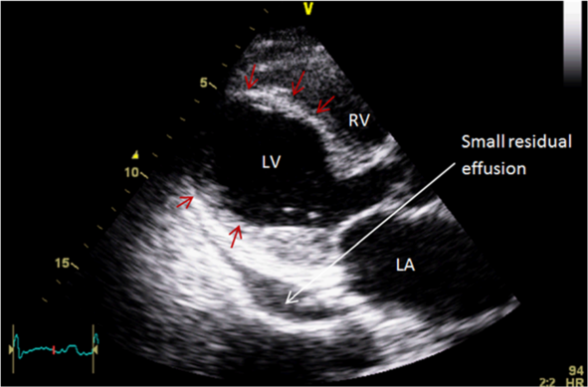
Parasternal long view post pericardiocentesis demonstrating apical ballooning (red arrows) as a result of apical and peri-apical akinesis
